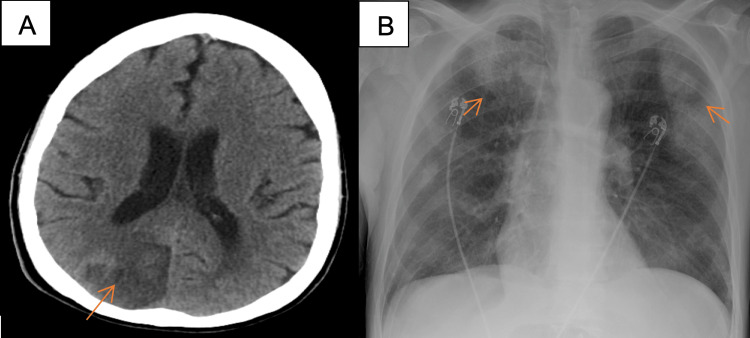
Non-contrast CT head and chest X-ray (A) Non-contrast CT head showing a recent-appearing right parieto-occipital infarct; (B) chest X-ray showing multifocal hazy opacities.
